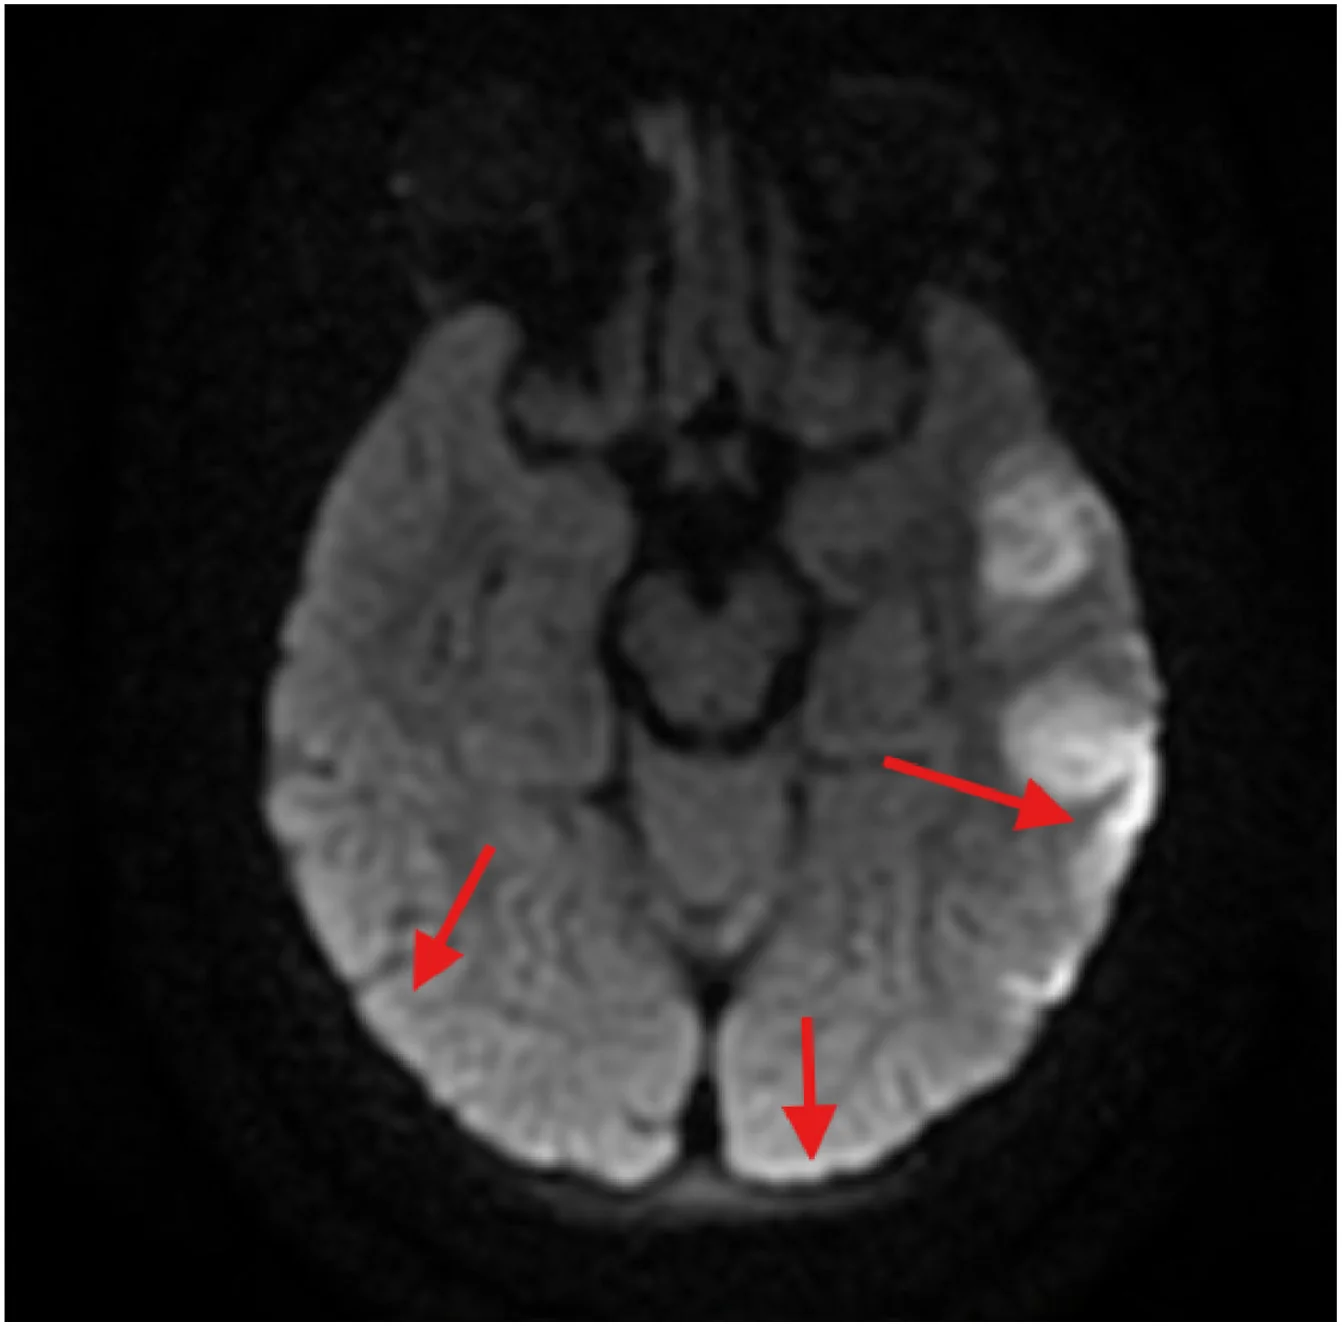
Axial diffusion-weighted imaging (DWI) of the brain. The images demonstrate areas of high signal intensity involving the bilateral parieto-occipital cortical regions with corresponding ADC reduction, consistent with acute ischemic infarction secondary to Moyamoya vasculopathy.
